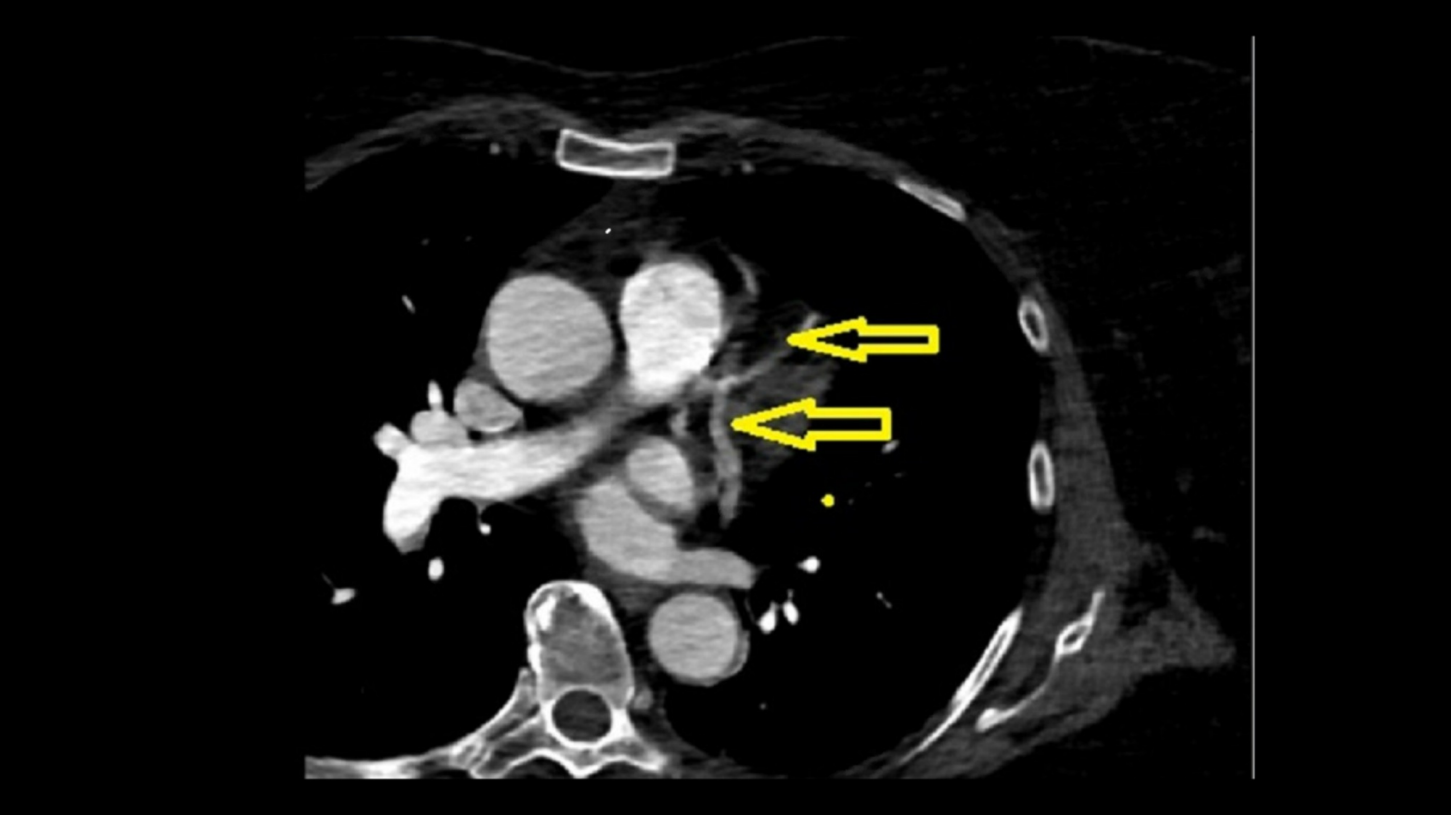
CT scan - view 1 The image shows the origin of RCA from mid-portion of LAD artery (top arrow points to LAD and bottom arrow points to RCA) CT: computed tomography; RCA: right coronary artery; LAD: left anterior descending
